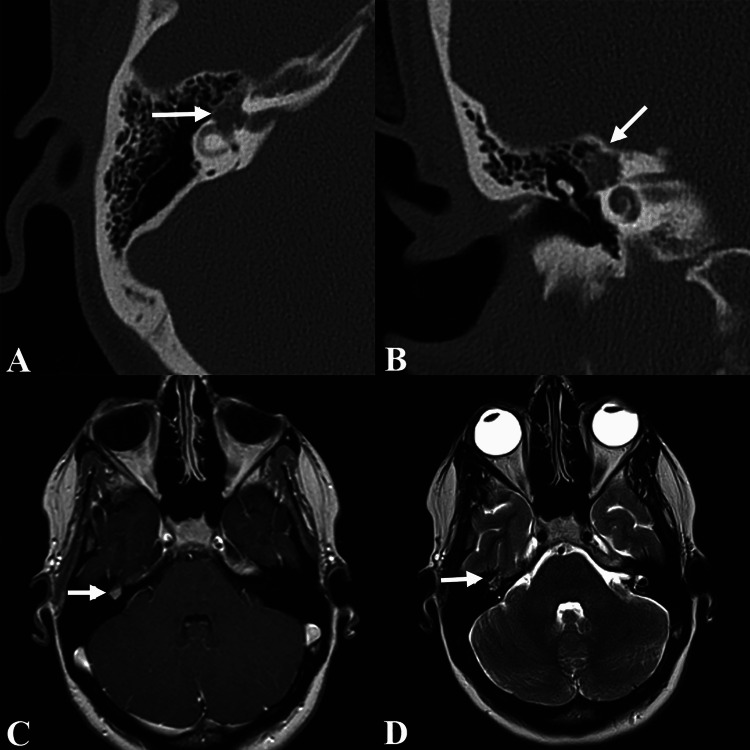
Imaging showing right perigeniculate mass. (A) Axial and (B) coronal computed tomography and (C) axial T1-weighted post-contrast and (D) axial T2-weighted magnetic resonance imaging displaying right perigeniculate mass (arrows).
